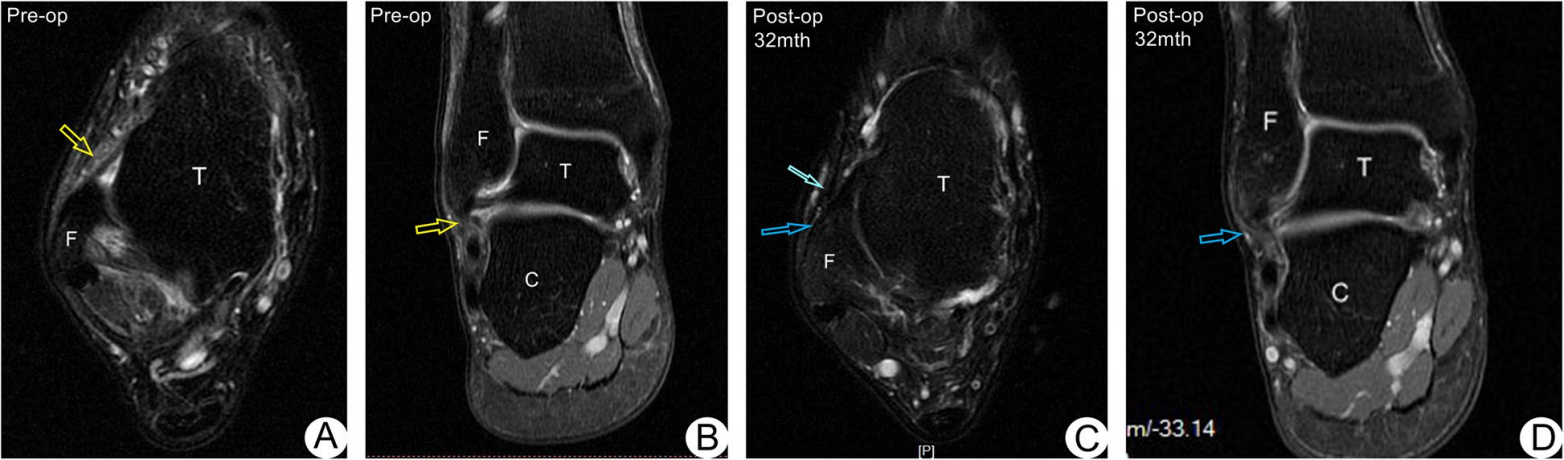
Female, 33 years old, had a sprained left ankle one year ago. a and b The axial and coronal T2-weighted images show the chronic lesion of the ATFL and CFL (*yellow arrows*). c and d The axial and coronal T2-weighted images show the appropriate regeneration of the ATFL and CFL (*bule arrows*) at 32 months postoperative. The reinforced IER was still attached at the lateral malleolus (*light bule arrows*). No degeneration of the subtalar joint was encountered
